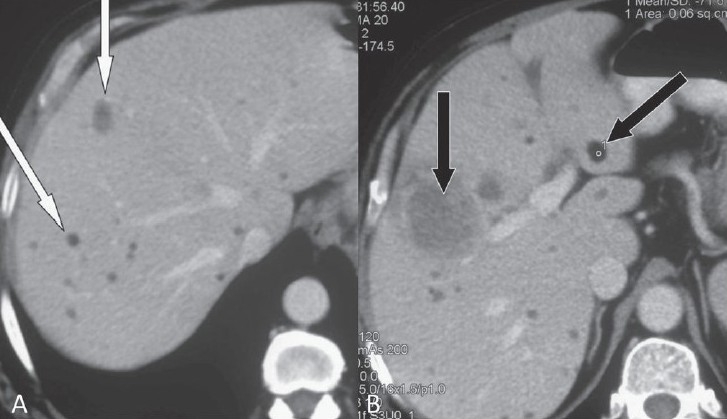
Axial contrast-enhanced CT scans show multiple, hypodense, focal lesions in both lobes of the liver with fatty attenuation (arrows)
